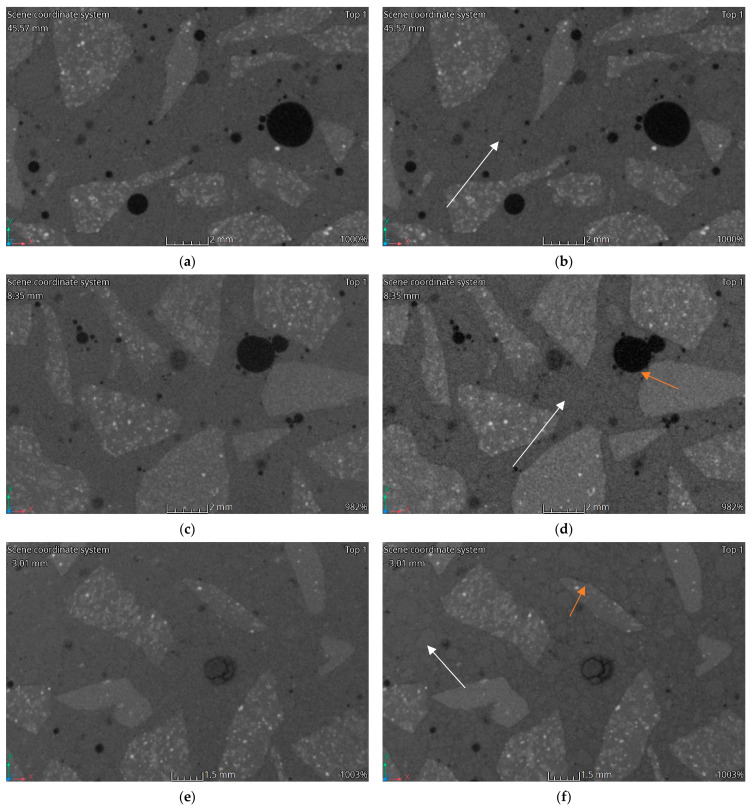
CT imaging results. Structural changes in three samples HPC: (a) 20 °C; (b) 200 °C; (c) 20 °C; (d) 400 °C; (e) 20 °C; (f) 600 °C.
